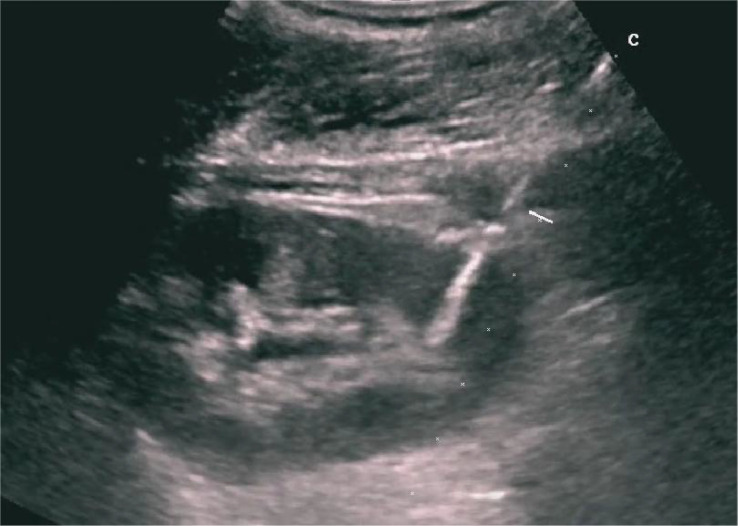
Ultrasound-guided display of the needle.
